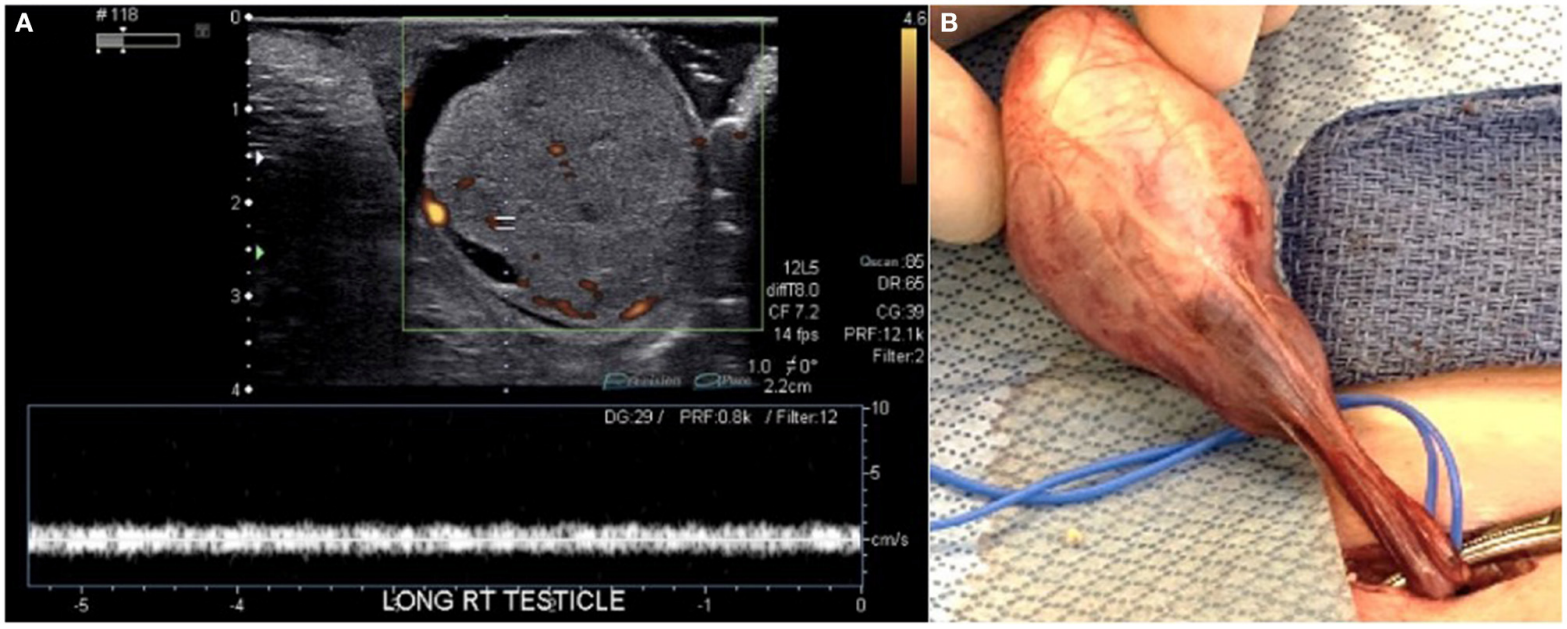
(A) Color Doppler ultrasonography showed an enlarged, heterogeneous, macronodular right testicle with cystic areas. Both arterial and venous flow were preserved, but hyperemia of the abnormal testis was present. (B) The testis and spermatic cord were accessed *via* an inguinal incision.
